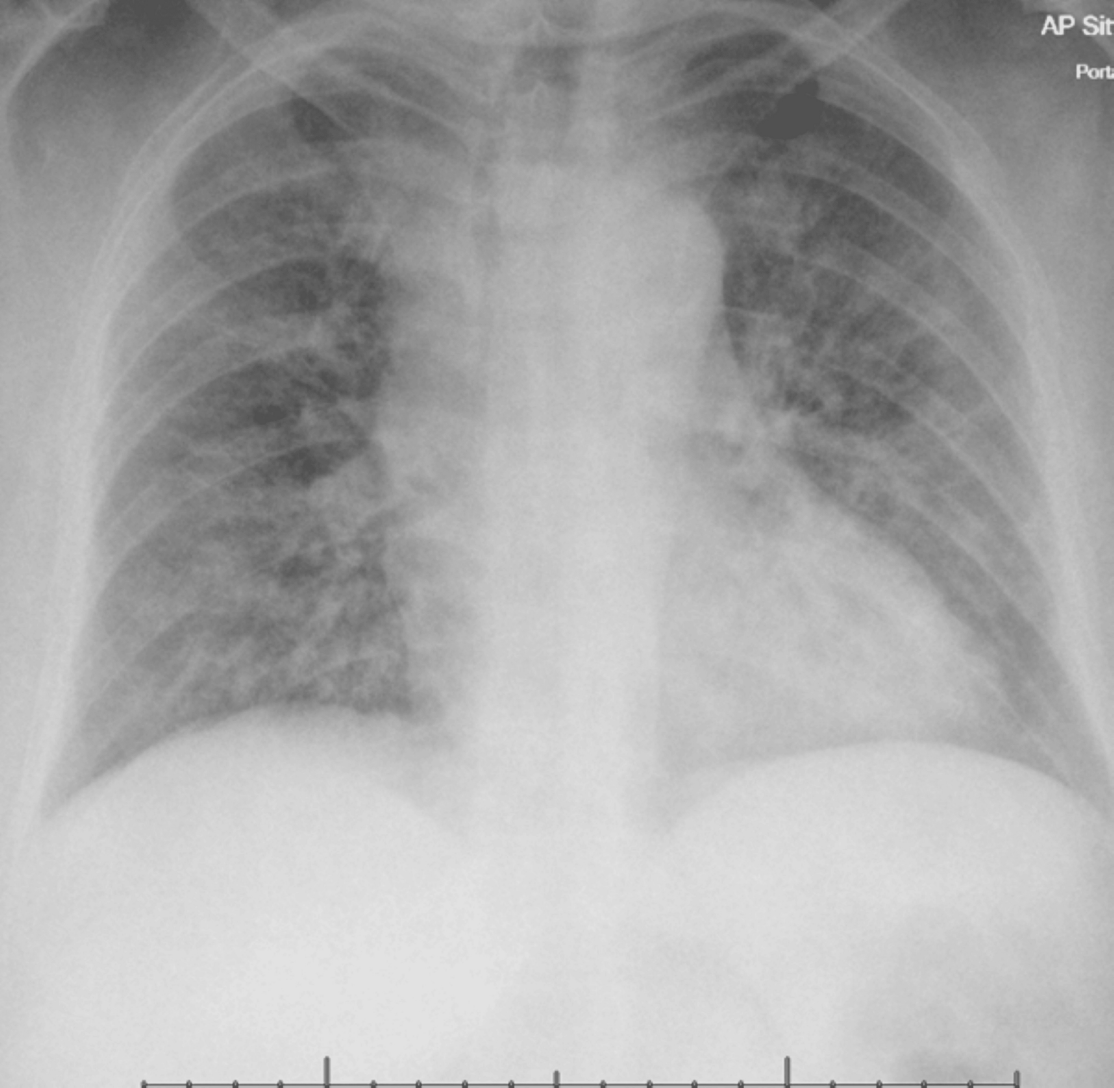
Chest radiograph demonstrates bilateral infiltrates.
